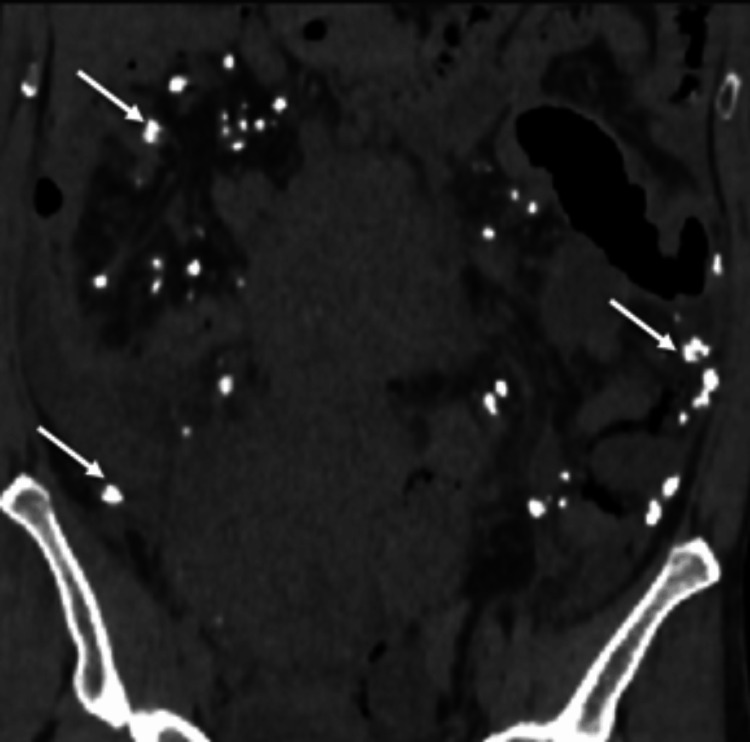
Coronal plain CT image showing multiple calcified mesenteric deposits (white arrows) CT: computed tomography
